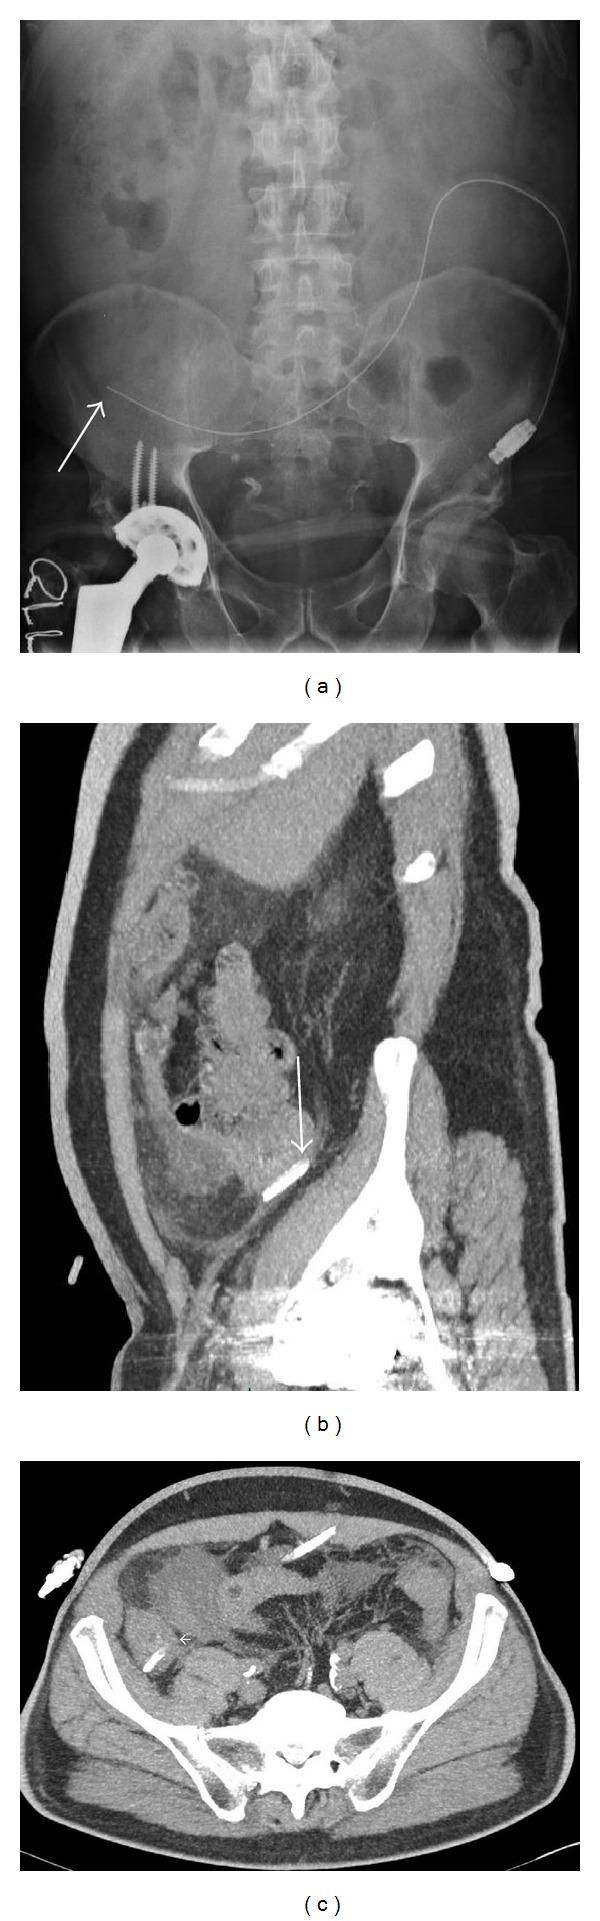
Radiologic findings. (a) Abdominal X-ray showing the PD catheter tip position in the right iliac fossa (white arrow). (b) Computerised tomography of the abdomen, showing the catheter tip resting in close contact with the pelvic wall and the colon (white arrow). (c) Computerised tomography of the abdomen, showing inflamed fat and bowel wall thickening (small white arrow).
